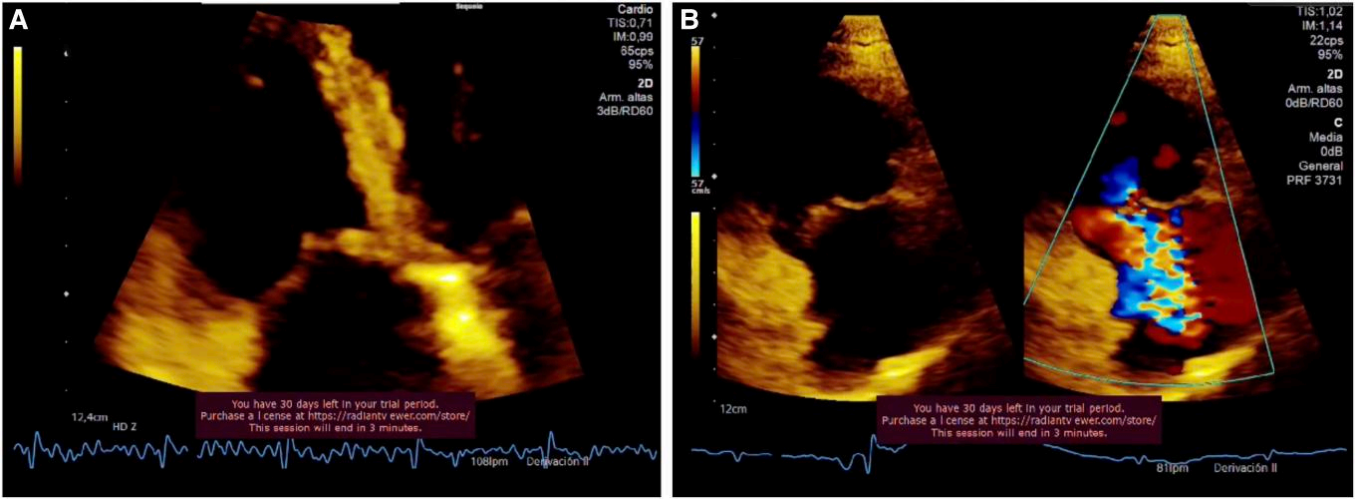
Transthoracic echocardiogram with a four-chamber view focusing on the right ventricle (*A*) and the right ventricular inflow tract (*B*). Thickening of the septal leaflet of the tricuspid valve with severe regurgitation is observed. RV, right ventricle.
